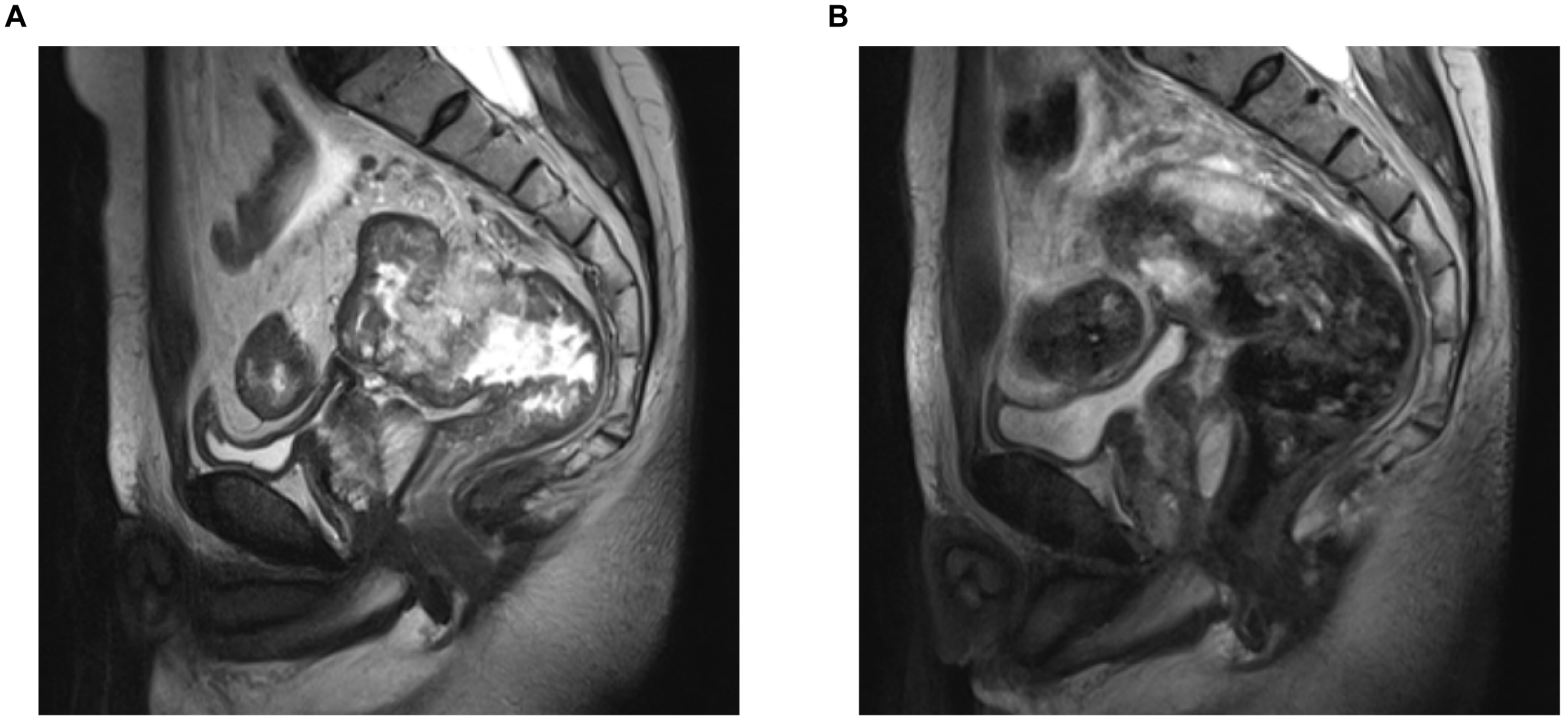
Pelvic MRI of the patient pre- and post-NAT. (A) Pre-NAT. (B) Post-NAT. NAT, neoadjuvant therapy.
